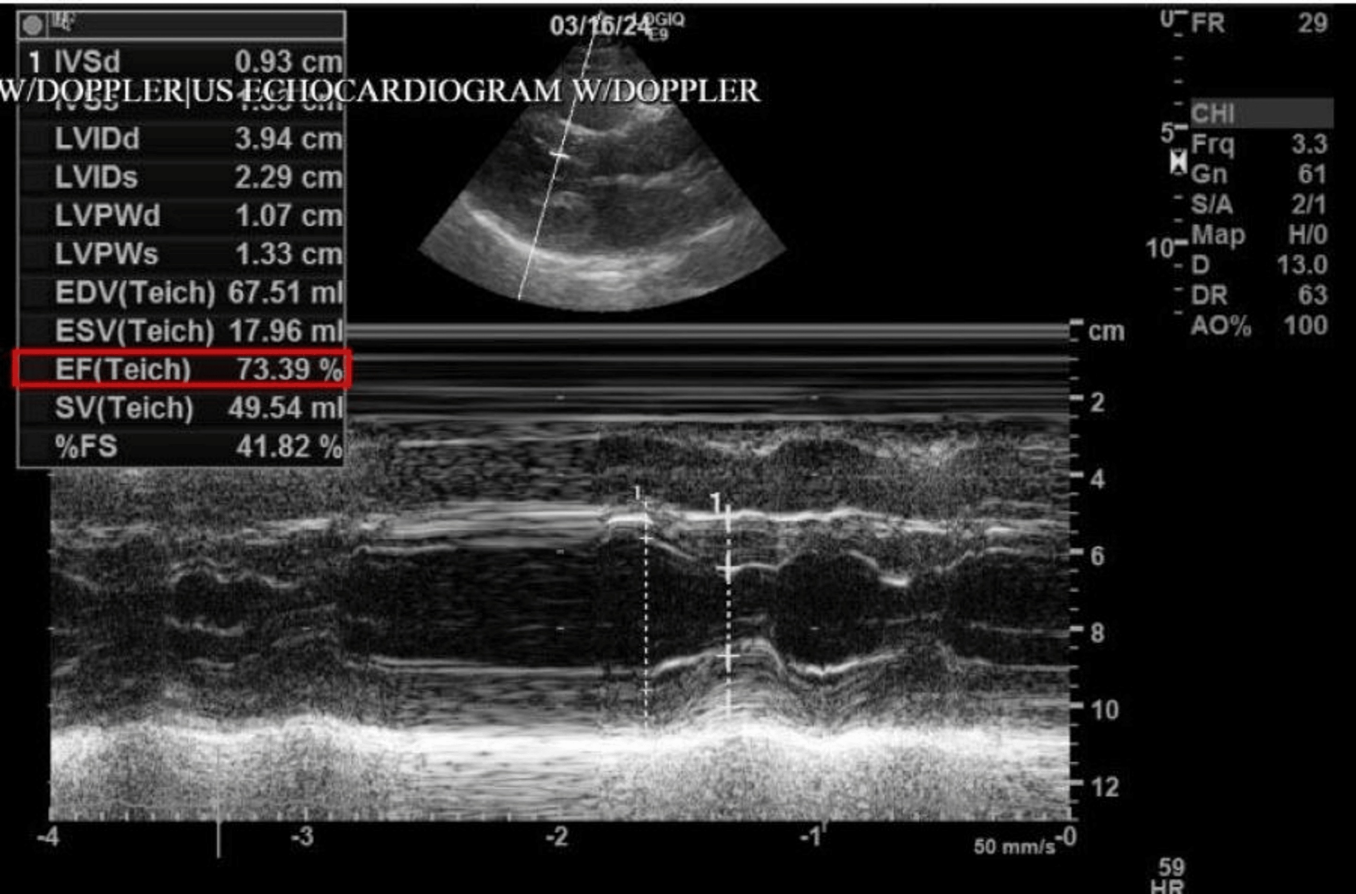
Transthoracic echocardiogram M-mode of the left ventricle showing preserved contractility of the LV. Preserved systolic function, indicated by an LVEF within the 54-74% range (red rectangle). LVEF, left ventricular ejection fraction
